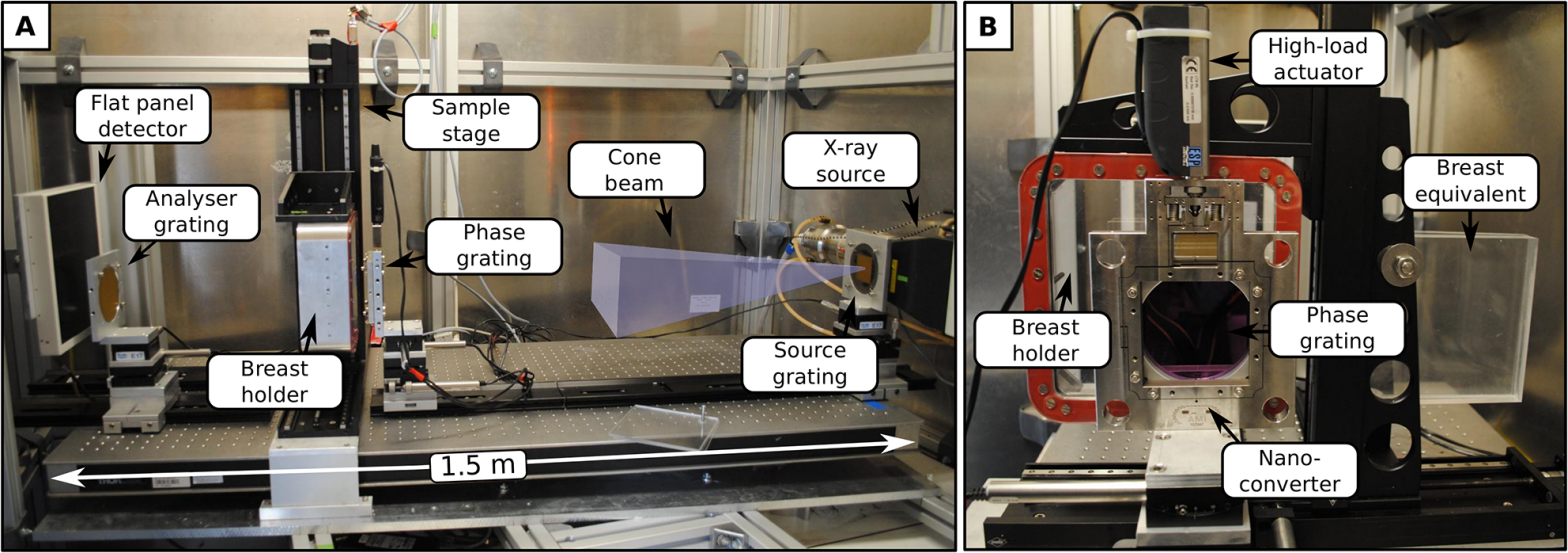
Laboratory X-ray phase-contrast mammography setup. (A) Longitudinal view of the revised, laboratory X-ray phase-contrast mammography setup with a dose-saving arrangement of phase grating and sample holder. A compact system length, rotating X-ray anode and conventional flat panel detector met the design criteria of clinical mammography systems. (B) Close-up view in the direction of the X-ray beam showing the phase-stepping instruments and breast equivalent incorporated for flat-field measurements. The combination of high-load actuator and nano-converter enables image acquisition times within 12 seconds.
